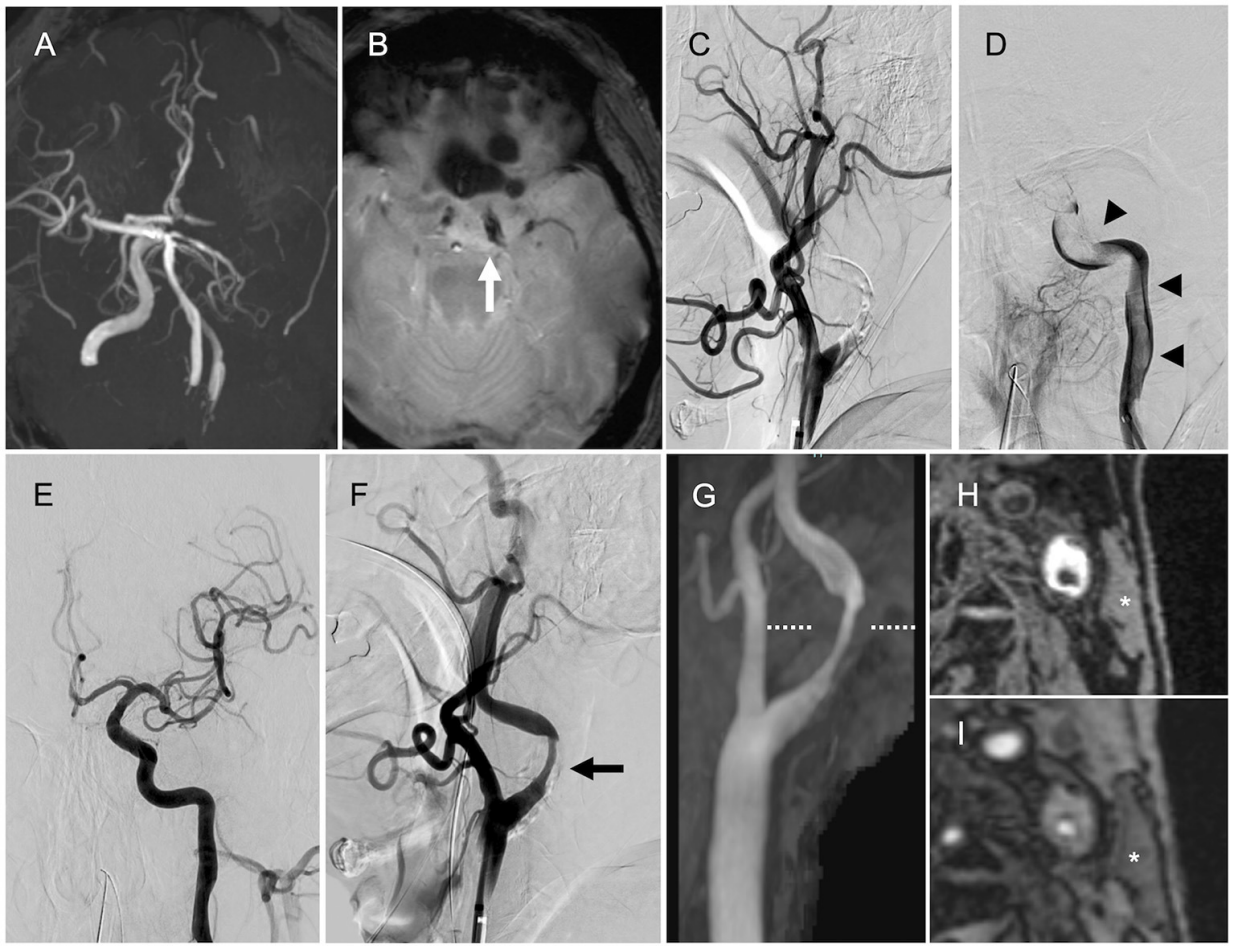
Magnetic resonance imaging (MRI) and angiography findings. (A) Magnetic resonance angiography (MRA) showing the left internal carotid artery (ICA) occlusion. (B) T2*-weighted MRI showing the susceptibility vessel sign at the top of the ICA (*arrow*). (C) Left internal carotid angiography showing the left proximal ICA occlusion (lateral view). (D) Angiography via the aspiration catheter in the cervical ICA showing a continuous filling defect (*arrowheads*) from the cervical ICA to the intracranial ICA (anteroposterior view). (E) Final angiography showing successful reperfusion (anteroposterior view). (F) Final angiography showing the left ICA stenosis (*arrow*, lateral view). (G) Neck MRA showing the left ICA stenosis. (H,I) Magnetization-prepared rapid gradient-echo image and maximum intensity projection images from time-of-flight MRA (level of the dotted line) showing high signal intensity in the plaque compared with the sternocleidomastoid muscle (*asterisk*).
